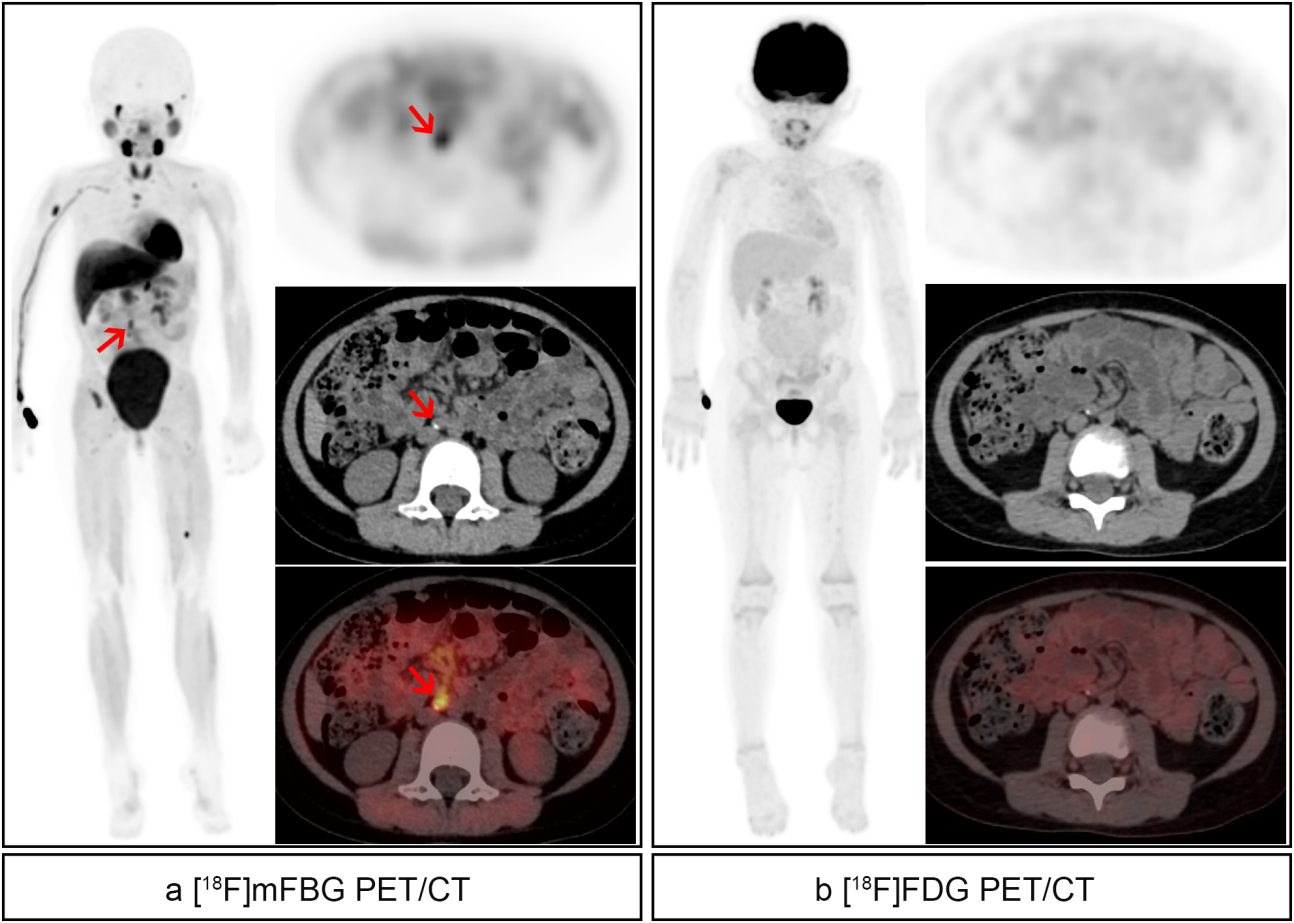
A 3-year-old girl suffered high-risk right retroperitoneal neuroblastoma with bone metastases and underwent resection of lesions followed by 8 cycles of chemotherapy. Post-therapy images were acquired. (a) [^18^F]mFBG images. Pathological uptake (red arrows) with SUVmax 5.1 and TBR 4.9 was noted in the abdominal paraaortic lymph nodes, and detailed showed in axial images. And residual activity of mFBG was found in bone/bone marrow in multiple parts of the body. (b) [^18^F]FDG images. No pathological uptake was found.
